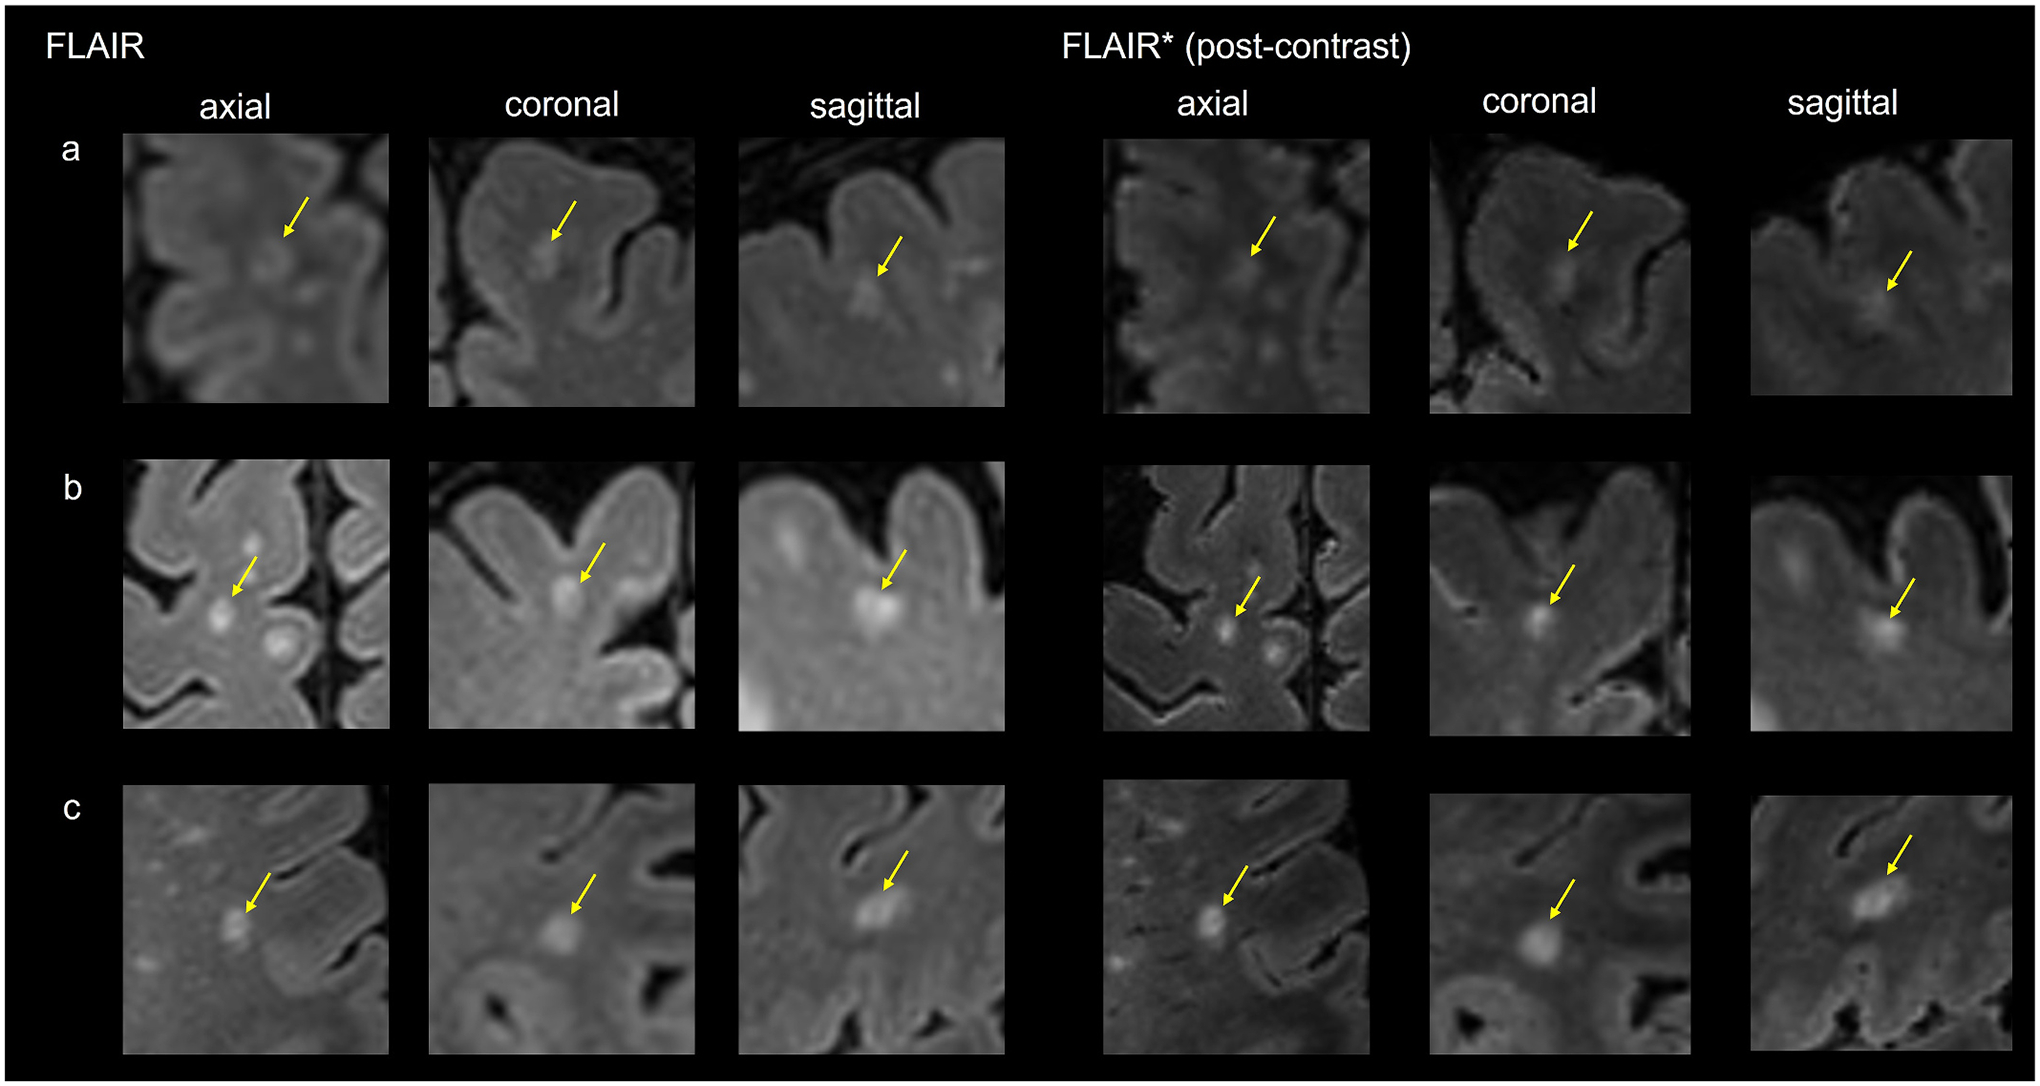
Examples of false positive findings on FLAIR, as characteristic central hypointensity was absent on post-contrast FLAIR* (a,b), or the lesion demonstrated multiple peripheral veins which were not evident on FLAIR (c).
